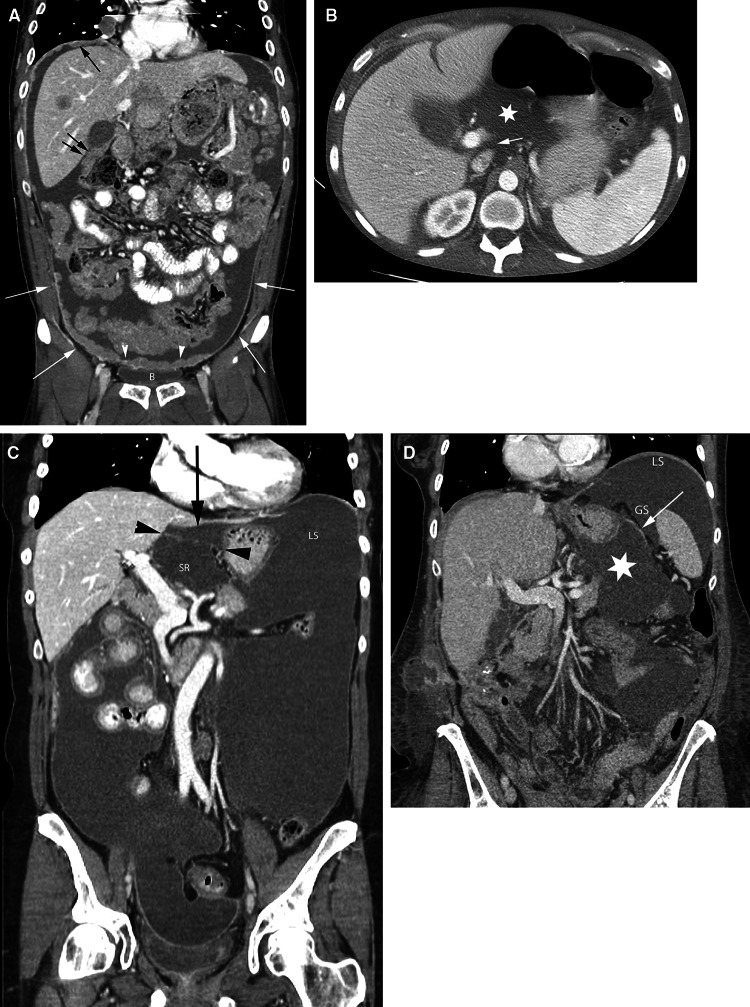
Peritoneal cavity fluid flow and recesses. A coronal CT image shows peritoneal carcinomatosis demonstrating the peritoneal recesses. Tumor in the right subdiaphragmatic recess (*black arrow*) and Morison’s pouch (*double black arrows*). Tumor (*arrowheads*) is also seen along the peritoneal reflection over the bladder (B). White arrows show continuity between the paravesical and paracolic recesses. B Axial CT image in a different patient shows the epiploic foramen (*arrow*) between the portal vein and inferior vena cava and fluid in the lesser sac (*asterisk*). C coronal CT image in a different patient shows fluid in the superior recess of the lesser sac (SR) and in the gastrohepatic recess (*black arrow*) separated by the gastrohepatic ligament (*black arrowheads*). Fluid in the gastrohepatic recess communicates with fluid in the left subphrenic space (LS). D coronal CT image in a different patient shows fluid in the lesser sac (*asterisk*) and in the gastrosplenic recess (GS) separated by the gastrosplenic ligament (*arrow*). There is continuity between the gastrosplenic and left subphrenic (LS) recesses.
